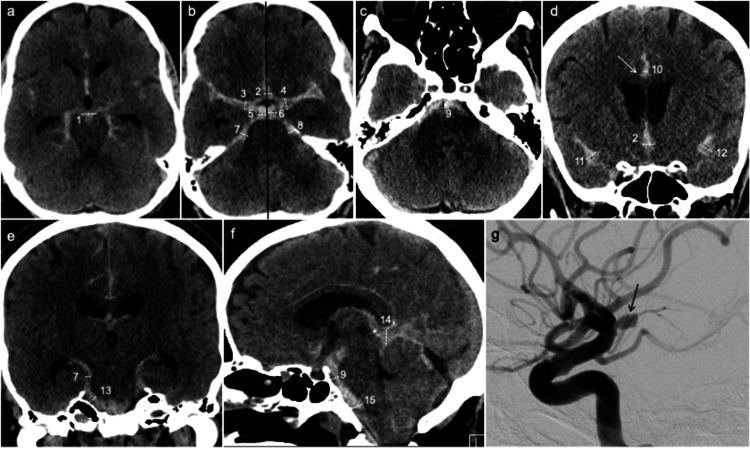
SAH from a ruptured aneurysm. CT scans of a 58-year-old male with acute SAH from a ruptured right carotid terminus aneurysm. The numbers in parentheses denote the cisterns. (a) Axial CT showing grade III 10-mm-thick SAH in the interpeduncular cistern (1). (b) Axial CT. The black central line demarcates the right and left sides of the suprasellar cisterns. The image shows a grade III 8 mm SAH in the ACom cistern (2), grade II 6 mm SAH in the base of the right Sylvian cistern (3), grade III 8 mm SAH in the base of the left Sylvian cistern (4), grade III 8 mm SAH in the right suprasellar cistern (5), grade II 6 mm SAH in the left suprasellar cistern (6), grade II 5 mm SAH in the right ambient cistern (7), and grade II 5 mm SAH in the left ambient cistern (8). (c) Axial CT showing a grade III 8 mm SAH in the prepontine cistern (9). (d) Coronal CT showing a grade III 8 mm SAH in the ACom cistern (2), grade I 3 mm SAH in the interhemispheric cistern (10), grade III 8 mm SAH in the right lateral Sylvian cistern (11), and grade III 8 mm SAH in the left lateral Sylvian cistern (12). Thin extension of a SAH into sulci branching from the main cistern. This is not included in the measurement of SAH thickness (arrow). (e) Coronal CT showing a grade II 4 mm SAH in the right ambient cistern (7) and a grade II 5 mm SAH in the right cerebellopontine angle cistern (13). (f) Sagittal CT showing a grade III 8 mm SAH in the prepontine cistern (9), a grade III 9 mm SAH in the quadrigeminal cistern (14), and a grade III 7 mm SAH in the premedullary cistern (15). (g) DSA of the same patient of a selective lateral view of the right internal carotid artery shows an irregular, posteriorly projecting 4 mm saccular aneurysm at the right carotid terminus (arrow). SAH: subarachnoid hemorrhage; CT: computed tomography; ACom: anterior communicating artery; DSA: digital subtraction angiography
